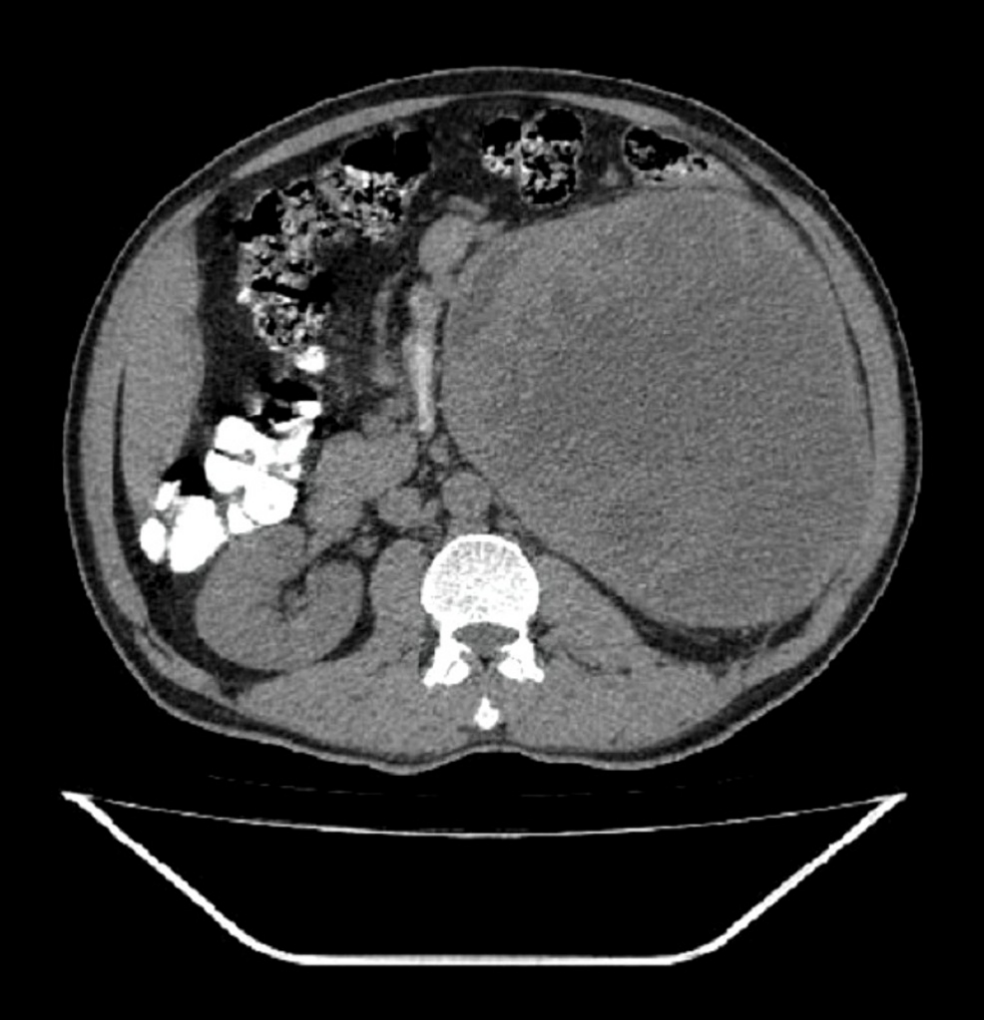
CECT of the abdomen showing a large solid cystic lobulated mass of the left kidney measuring 26 x 16cm in size with perilesional streakiness. CECT, contrast-enhanced computed tomography
